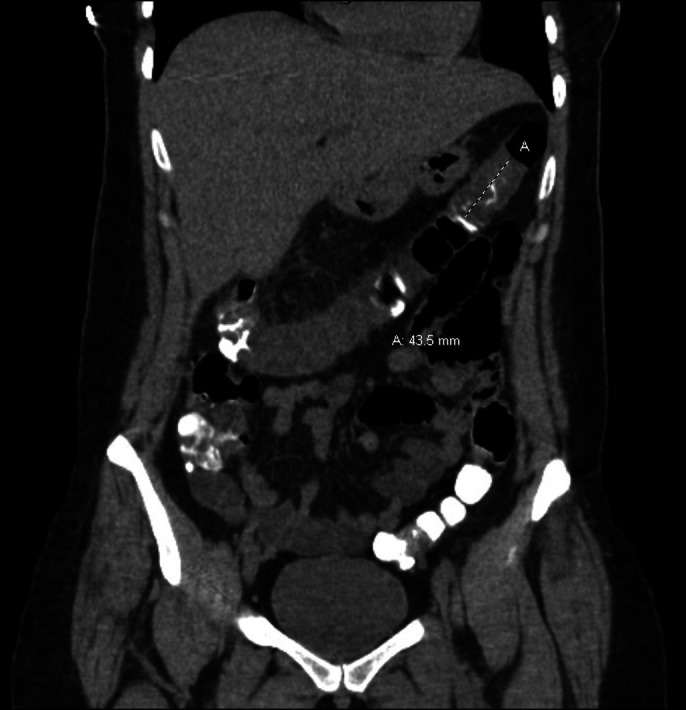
A day after treatment, computed tomography showing resolution of the intussusception.
